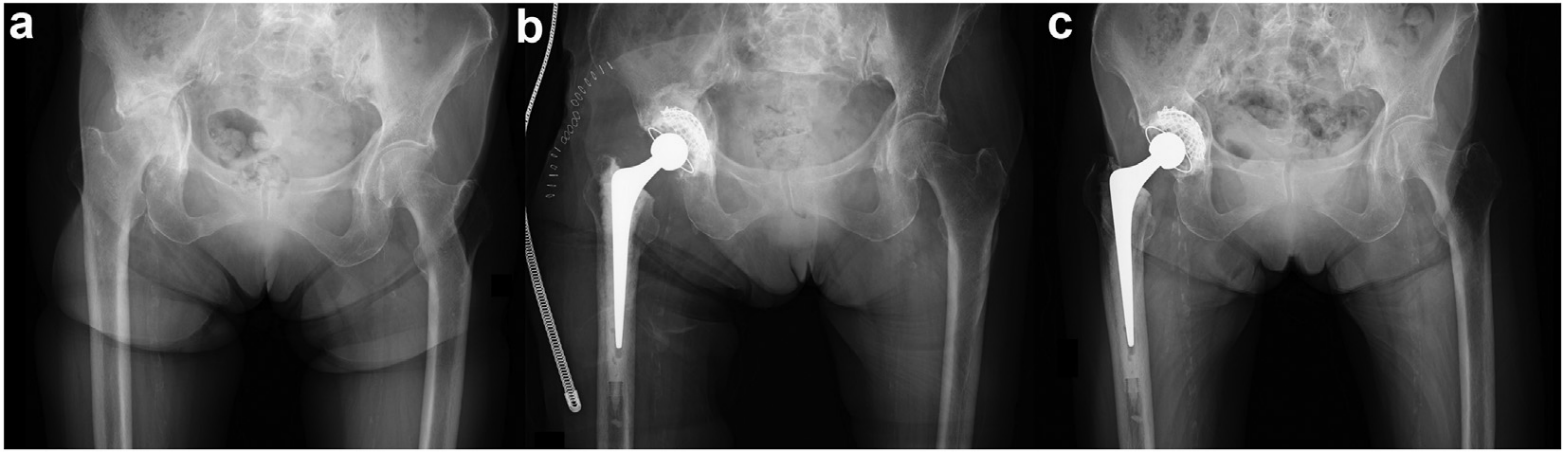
A case presentation (case 1). (a) Radiographic imaging at the patient’s first visit to our hospital showed a comminuted acetabular fracture with quadrilateral surface destruction as well as a femoral head fracture. Good callus formation on the acetabulum was evident. (b) Radiographic images after late THA with IBG 2 months after the initial trauma. (c) A radiographic image at the 3-year postoperative follow-up showing no component loosening or any subsidence of the acetabular cemented cup.
